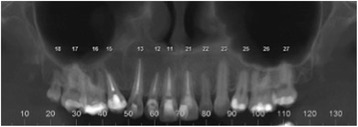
Panoramic image of computed tomography scan showing recurrence
